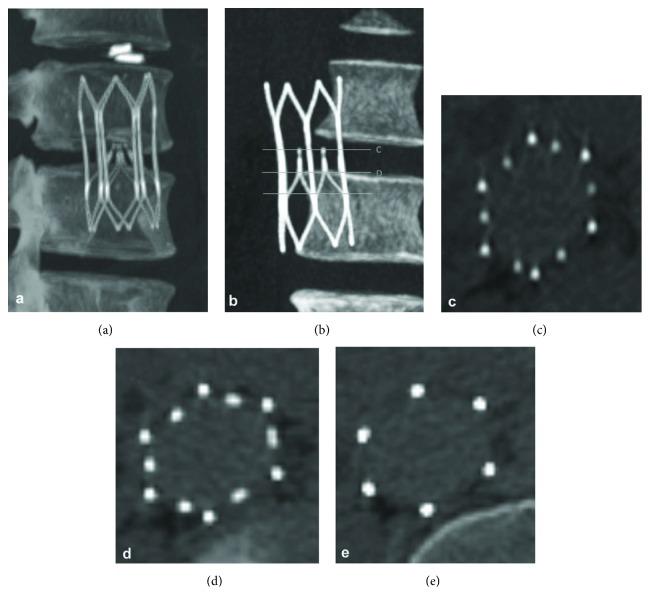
Computed tomography imaging of the Sentry bioconvertible IVC filter for a single patient. (a) Coronal image acquired as part of helical dataset 1 month after filter placement, showing the Sentry device in filtering configuration. (b) Coronal image at 6-month follow-up showing the device in bioconverted configuration. ((c), (d), (e)) Axial views of the bioconverted device at 6-month follow-up, with the images keyed to the coronal view in (b). Reprinted with permission from Dake et al. [60]
